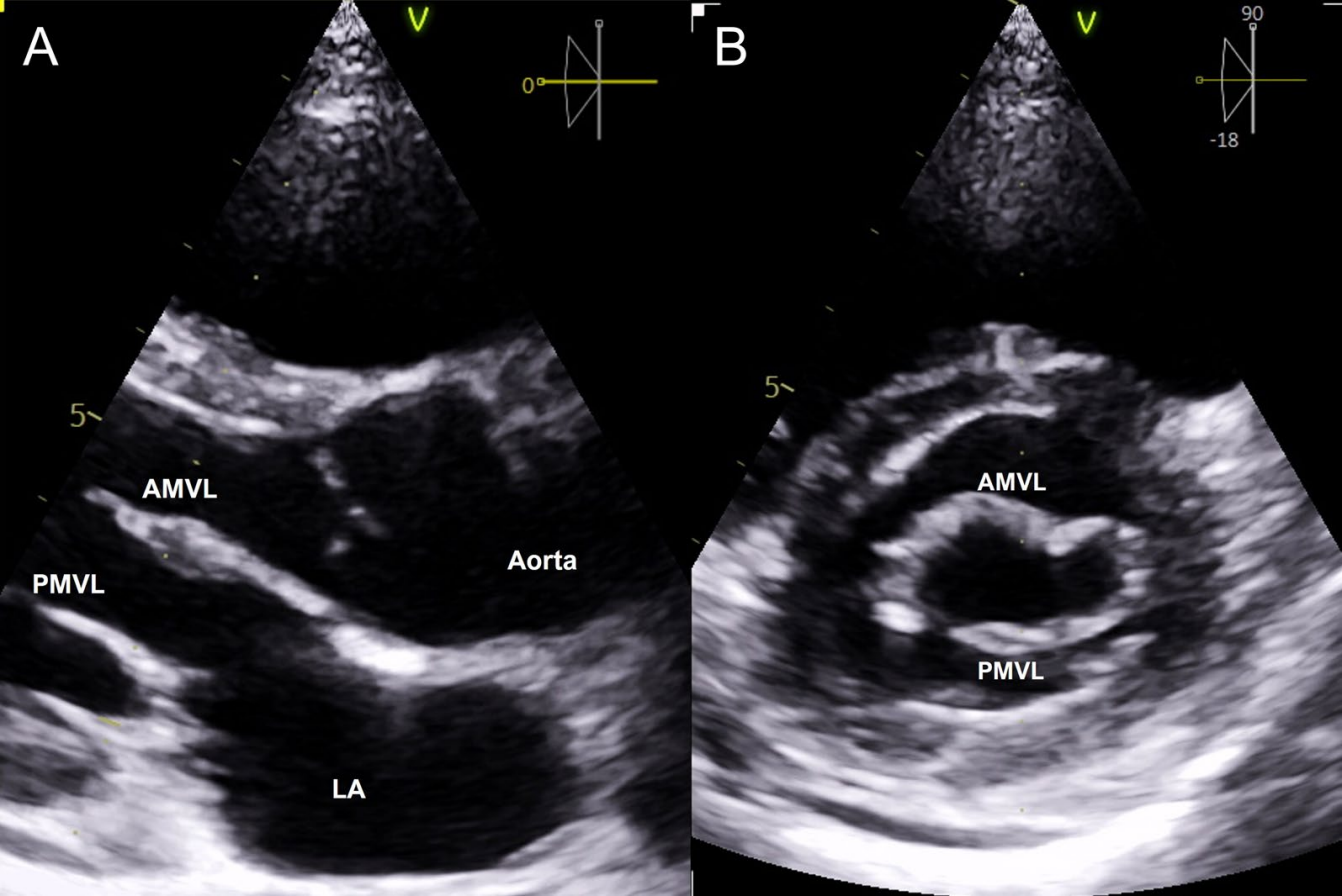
Sequential postoperative echocardiographic images. Legend: Panel A: 2D TTE parasternal long axis view showing intact AMVL and PMVL without any vegetations. Panel B: 2D TTE parasternal short axis view of the MV leaflets with no vegetations noted on the PMVL. 2D, two-dimensional; TTE, transthoracic echocardiography; AMVL, anterior mitral valve leaflet; PMVL, posterior mitral valve leaflet; MV, mitral valve
